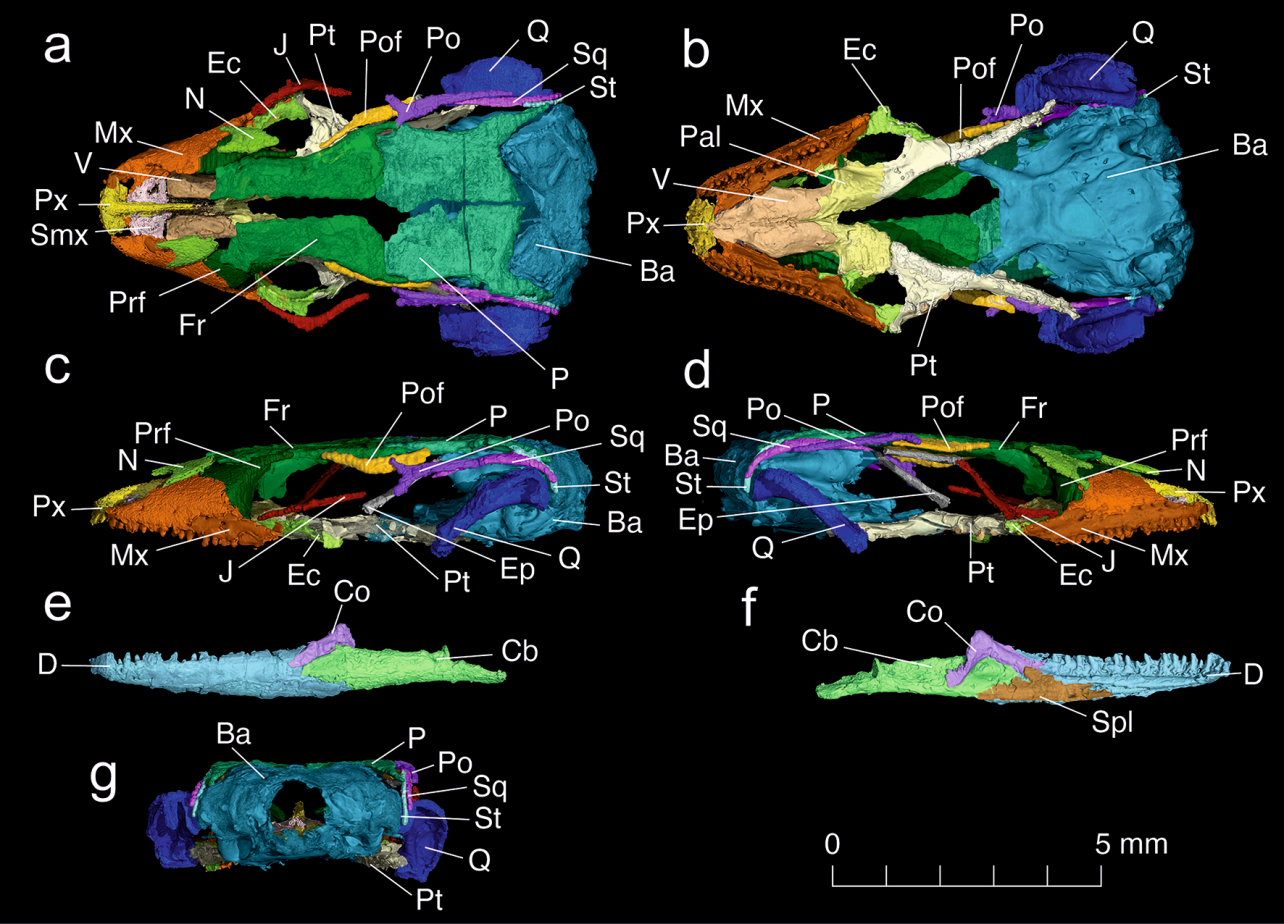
Skull of *Retinosaurus hkamtiensis* (GRS 29689) based on X-ray microcomputed tomography (synchrotron data). Individual bones segmented using VGSTUDIO MAX (Volume Graphics GmbH), rendering each bone as a volume. Cranium in dorsal (a), ventral (b), left lateral (c), right lateral (d), and posterior (g) views, Jaw in lateral (e) and medial (f) views. *Ba* braincase, *Cb* compound bone, *Co* coronoid, *D* dentary, *Ec* ectopterygoid, *Ep* epipterygoid, *Fr* frontal, *J* jugal, *Mx* maxilla, *N* nasal, *P* parietal, *Pal* palatine, *Po* postorbital, *Pof* postfrontal, *Prf* prefrontal, *Pt* pterygoid, *Px* premaxilla, *Spl* splenial, *Q* quadrate, *Smx* septomaxilla, *Sq* squamosal, *St* supratemporal, *V* vomer.
